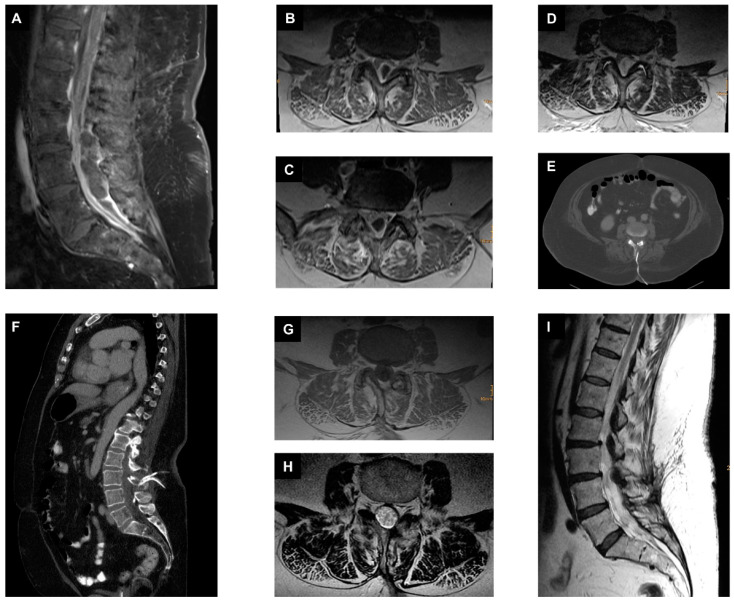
Management of ISEE with epidural suction–irrigation drainages. (A): A preoperative sagittal fat-saturated contrast-enhanced T1-weighted MR image of the lumbar spine shows isolated spinal epidural empyema in the dorsal part of the spinal canal at the level of L3 to S1. (B–D): Different preoperative axial T1-weighted contrast-enhanced MR images of the lumbar spine at the level of L3, L4, and L5. (E,F) Postoperative axial and sagittal reformatted CT images showing the previously placed suction–irrigation drainages cranially and caudally in the spinal canal at the level of L3/L4. (G): Axial T1-weighted contrast-enhanced MR image at 10 weeks at the time of antibiotic discontinuation. (H): Axial T2-weighted MR image at 10 weeks at the time of antibiotic discontinuation. (I): Sagittal T2-weighted MR image at 10 weeks at the time of antibiotic discontinuation.
